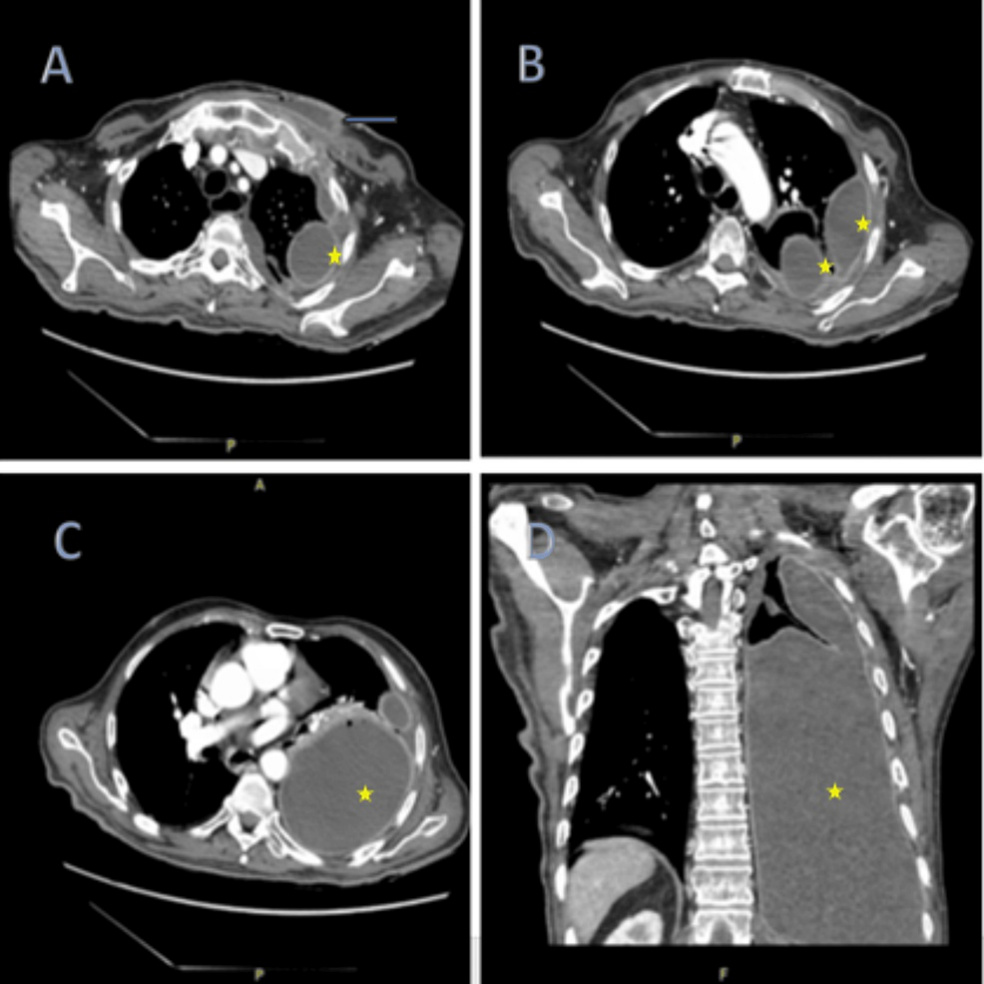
CT chest showing left-sided loculated pleural effusion and soft tissue swelling at the sternoclavicular level. Axial (A, B, C) and coronary (D) planes. Blue arrow: soft tissue swelling on the left chest wall, Yellow stars: loculated pleural effusion
